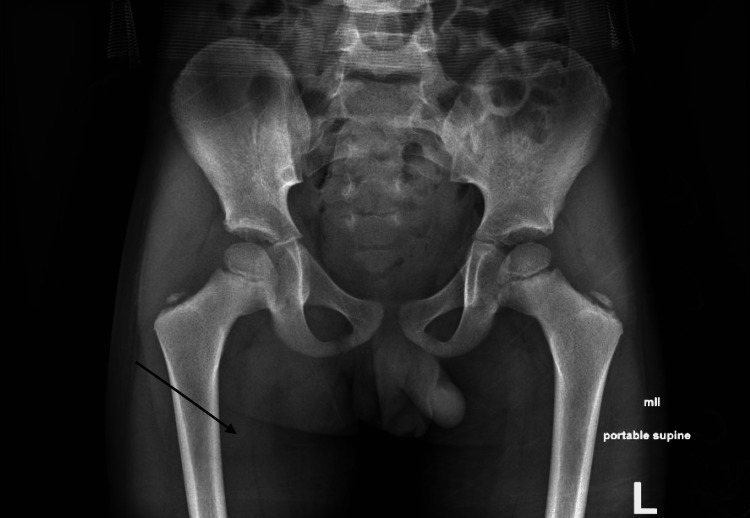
Plain radiograph of the pelvis Anteroposterior radiograph of the pelvis and right femur obtained at the initial emergency department visit without a visible foreign body or osseous injury. The arrow marks the site of the puncture wound.
